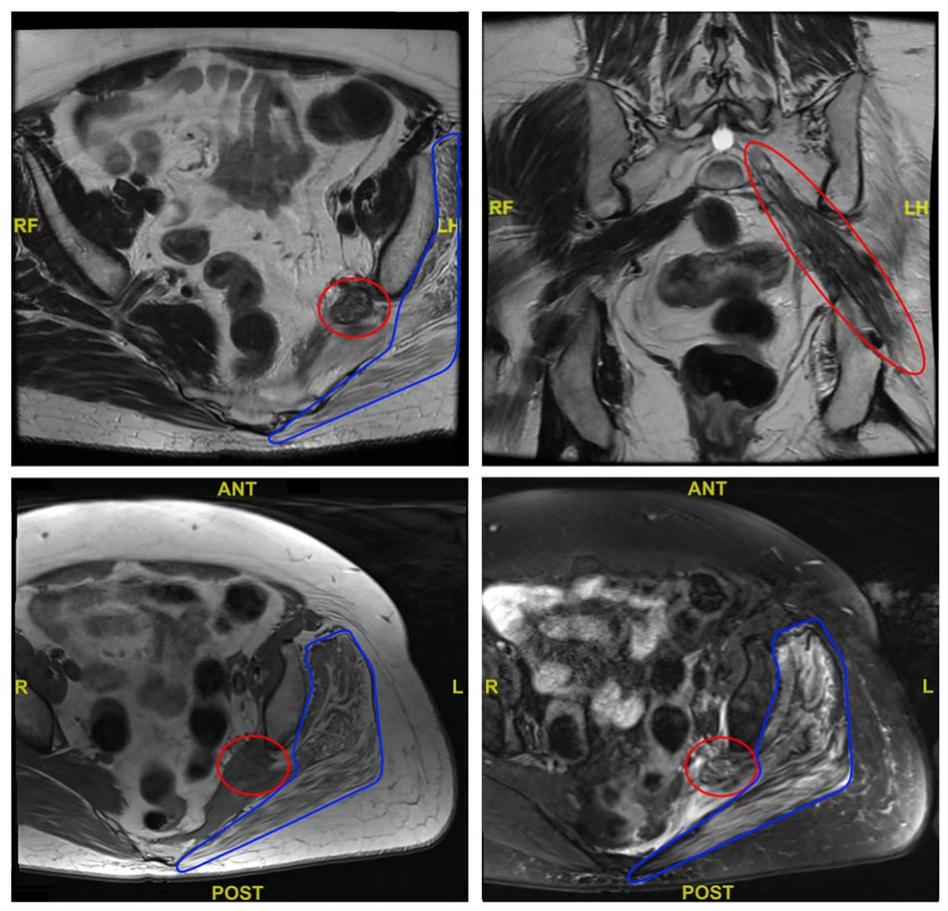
Selected axial and coronal views from MRI pelvis showing a thickened and diseased left sciatic nerve (red circle) and atrophied left glutei with fatty infiltration (blue outline).
